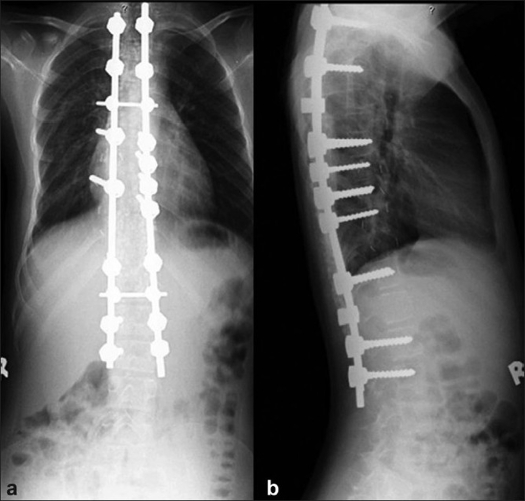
Post-operative anteroposterior (a) and lateral (b) radiographs of dorsal and lumbar spine depicting the deformity correction achieved by all screw construct
